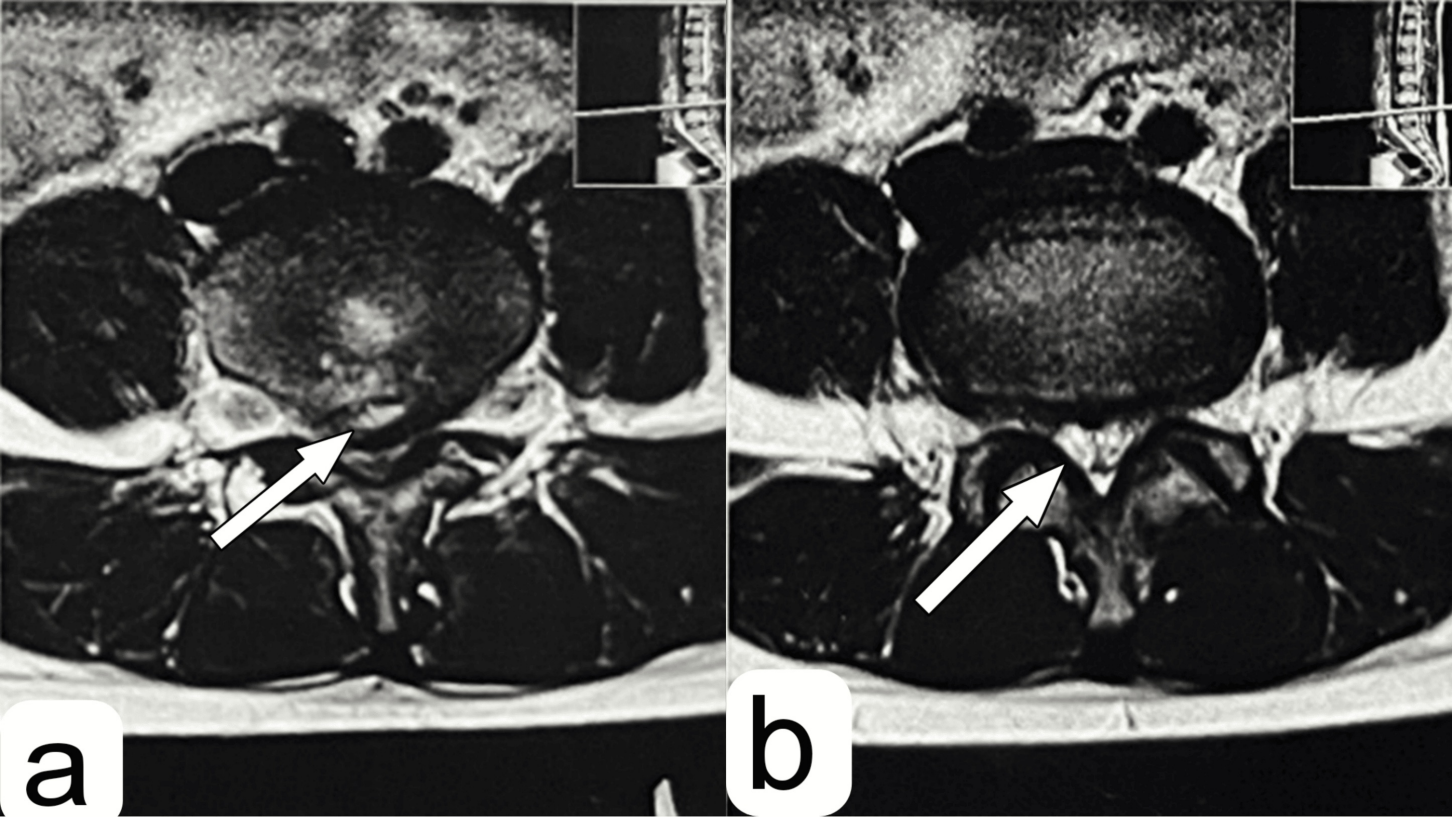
T2-weighted MRI axial sections of the L4-L5 level T2-weighted MRI axial cut showing (a) posterior ring apophyseal fracture fragment (white arrow) and (b) severe compromise of bilateral lateral recesses, ligamentum flavum hypertrophy with compression of bilateral L5 traversing, and abutment of bilateral L4 exiting nerve roots due to mild compromise of bilateral neural foramina (white arrow). MRI: magnetic resonance imaging
